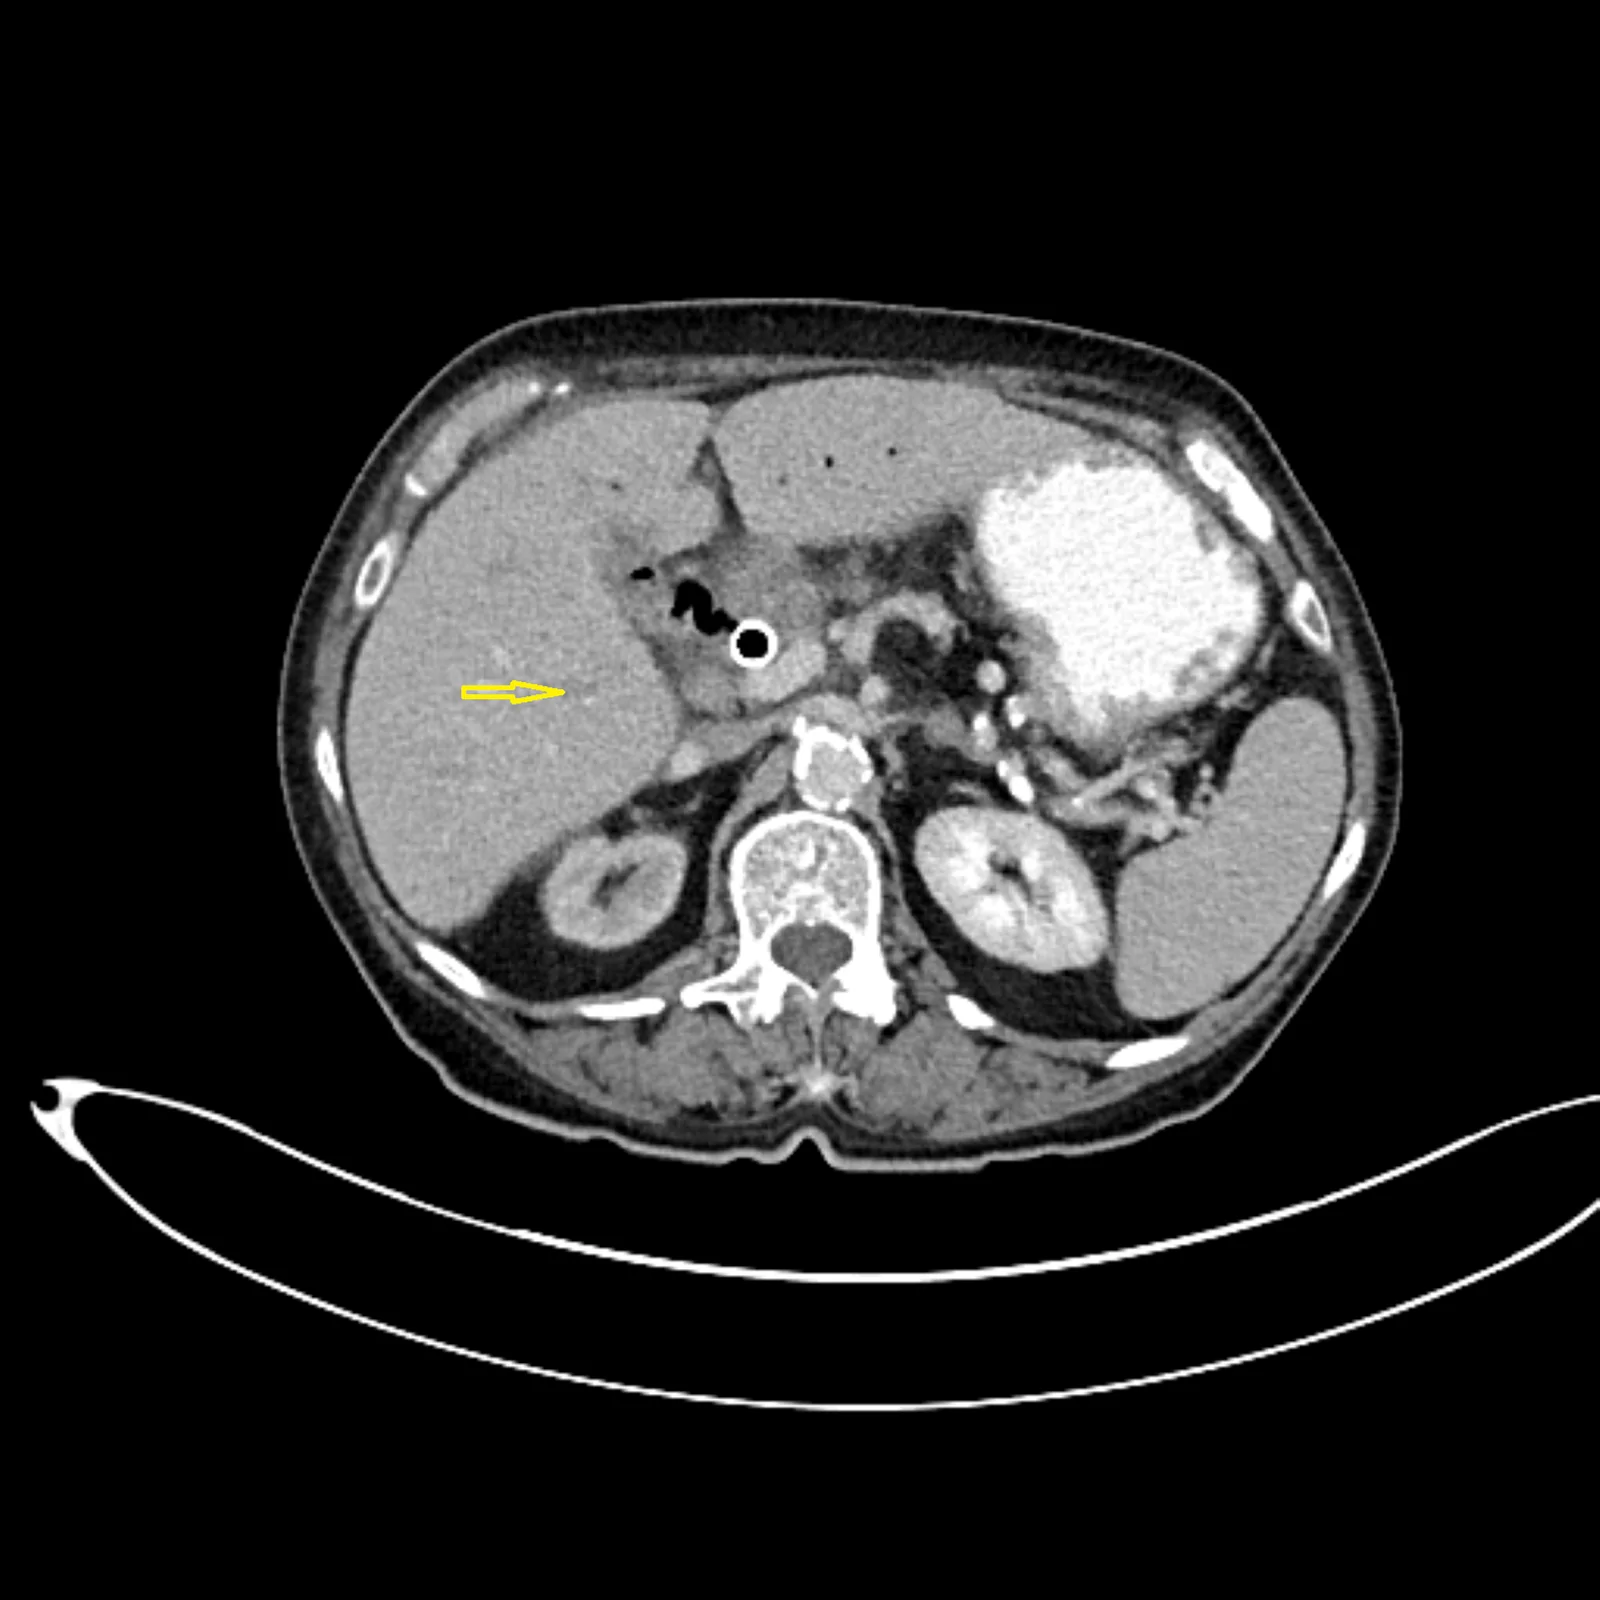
Cross-section of CT abdomen with contrast, the yellow arrow pointing towards multiple liver hypodensities consistent with metastatic disease.
